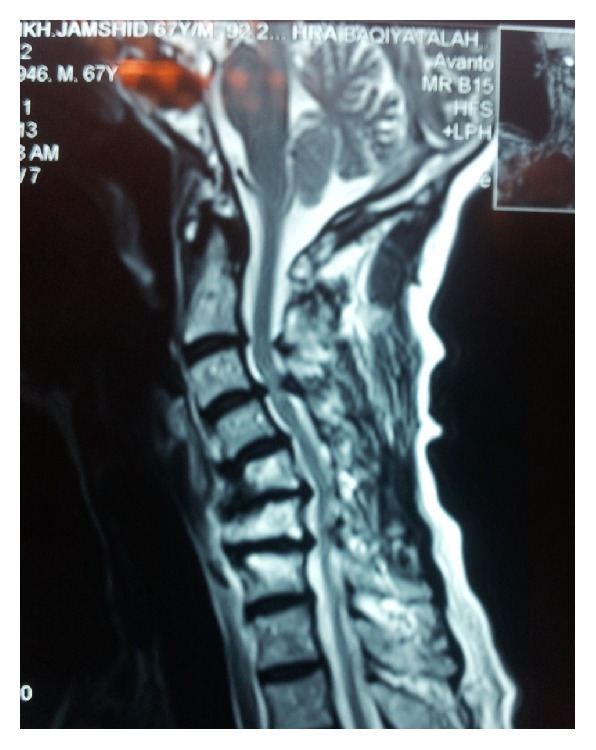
T2-weighted sagittal image in 2013, revealing aggravation spondylotic changes with moderate cord compression; note hyperintensity at C3-C4 level.
